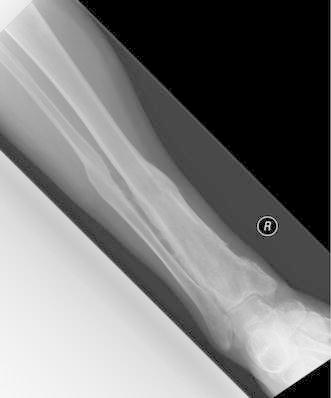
Plain radiograph of the right distal tibia following second stage bone grafting
